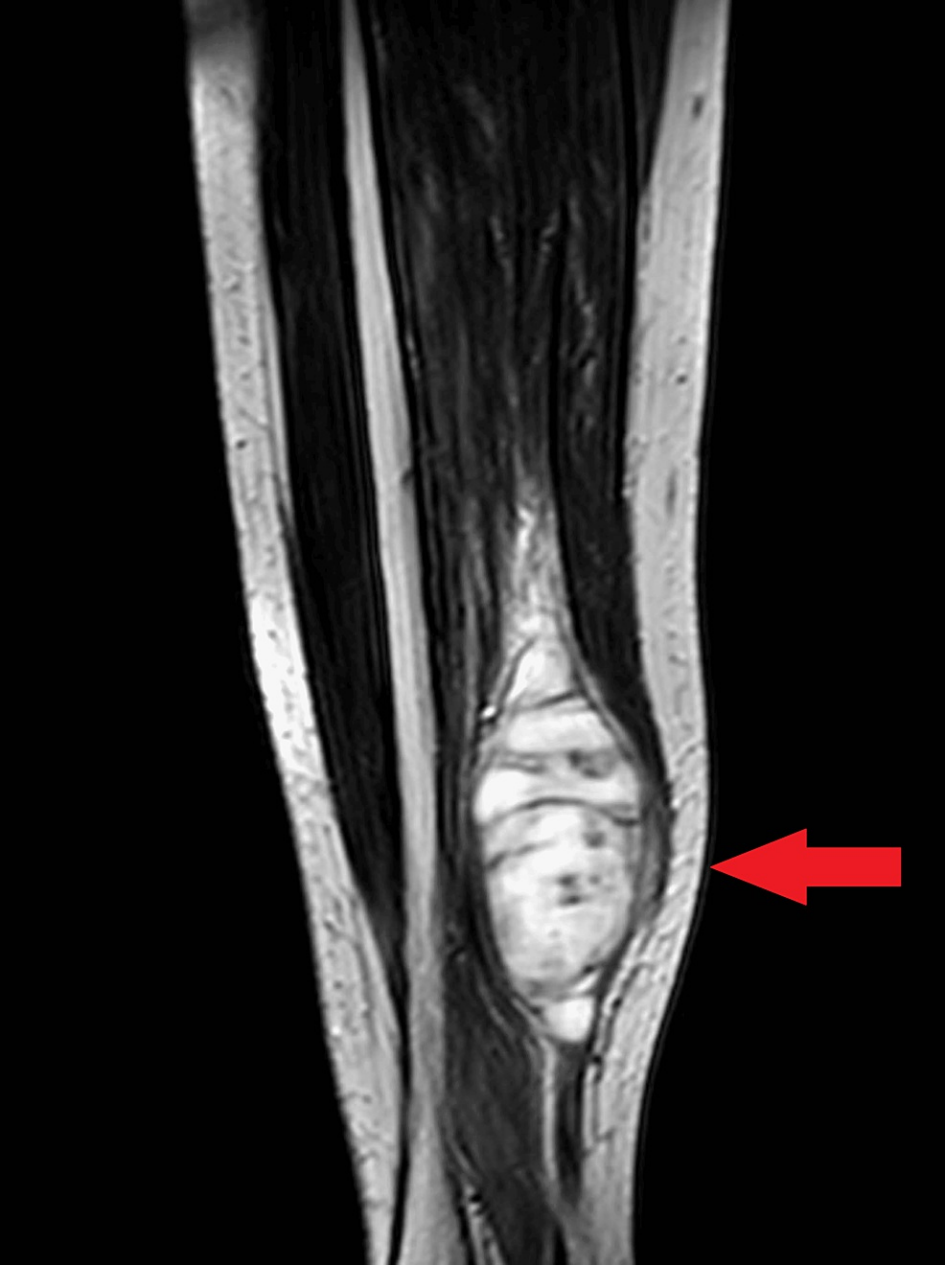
Contrast-enhanced MRI (coronal view) of the right leg showing a multi-lobulated spindle-shaped mass lesion with heterogeneous enhancement and altered signal intensity in the region of the knee joint
